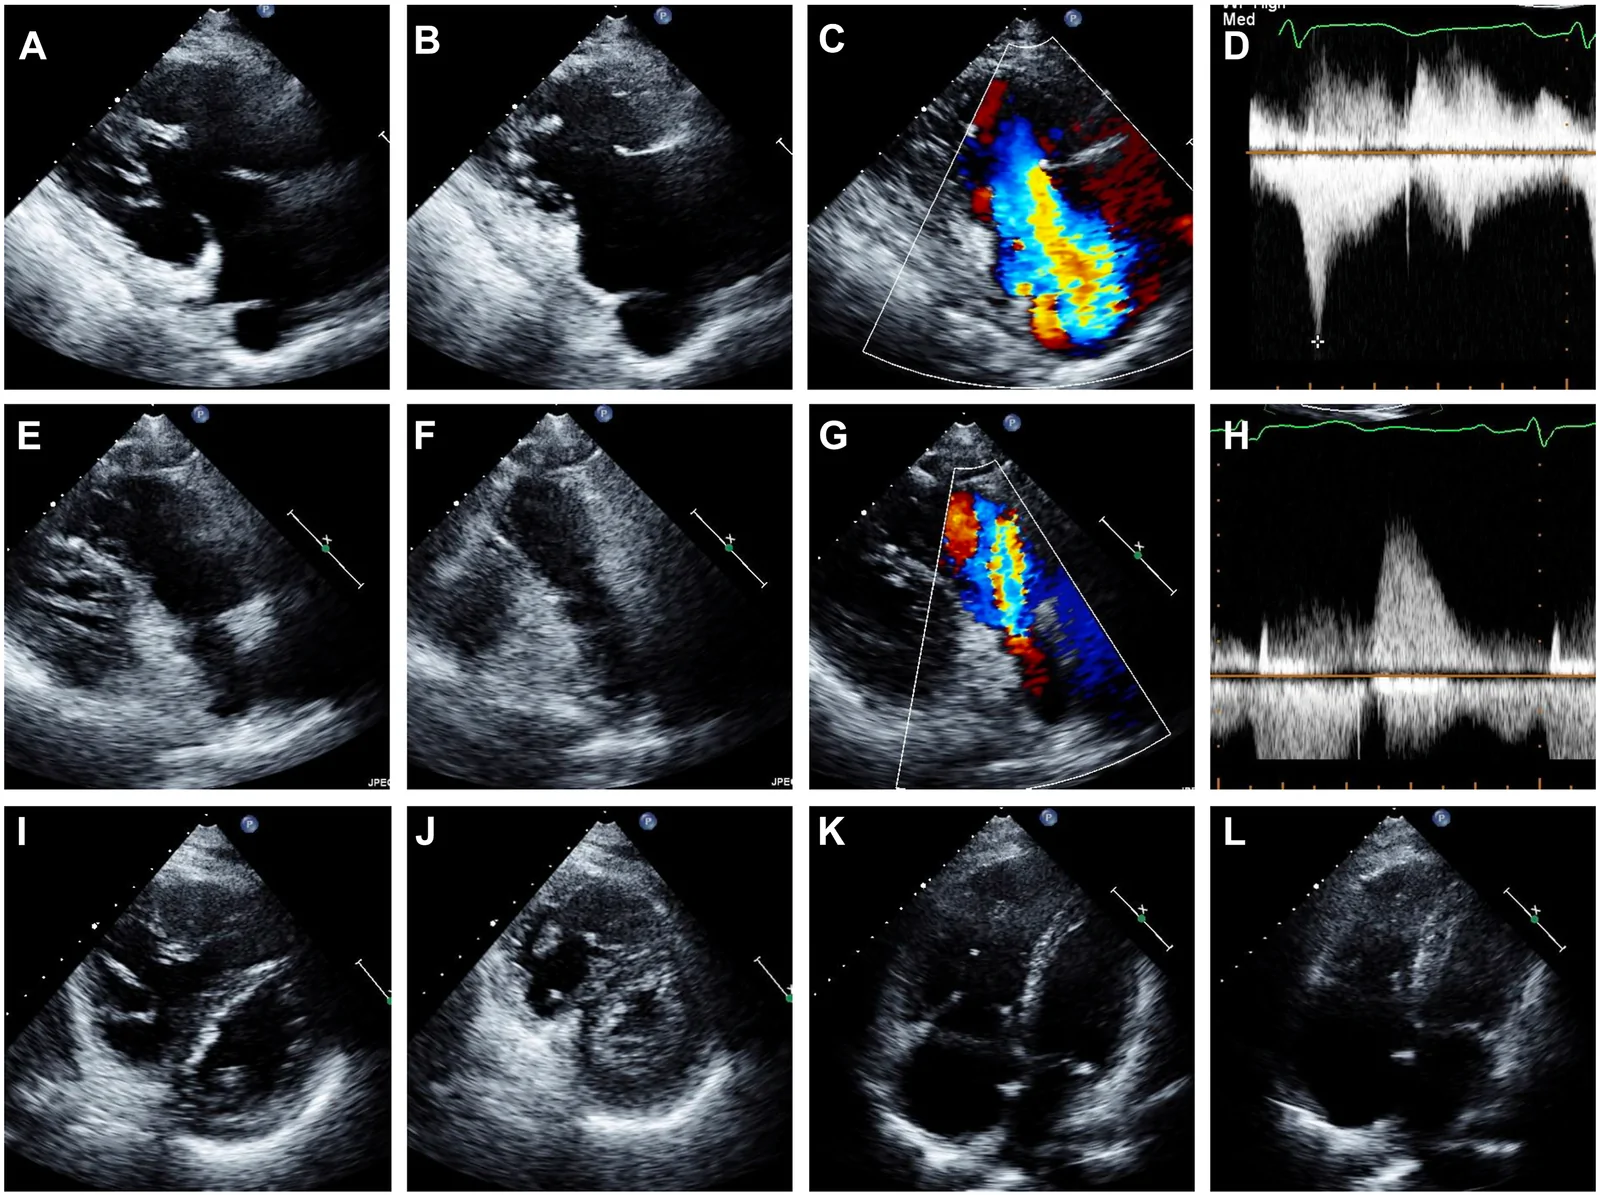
Echocardiographic findings of carcinoid heart disease. Representative transthoracic echocardiogram images of a patient in our cohort with carcinoid heart disease. Parasternal right ventricular (RV) inflow view shows fixed, retracted, and thickened tricuspid valve leaflets in (A) end-diastole and (B) end-systole. Evidence can be seen of severe tricuspid regurgitation based on (C) the large regurgitant jet area on colour Doppler and (D) the dense, triangular-shaped continuous wave Doppler signal in systole. Parasternal RV outflow view shows a similar appearance of the pulmonary valve in (E) end-diastole and (F) end-systole. Severe pulmonary regurgitation is suspected due to (G) the large regurgitant jet area on colour Doppler and (H) dense continuous wave Doppler signal with pressure half-time < 100 ms in diastole. Parasternal short-axis views show ventricular septal flattening in (I) end-diastole that is absent in (J) end-systole due to volume overload from severe right-sided valvular regurgitation. Similarly, apical 4-chamber views in (K) end-diastole and (L) end-systole show severe right atrial and RV enlargement from right-sided volume overload.
